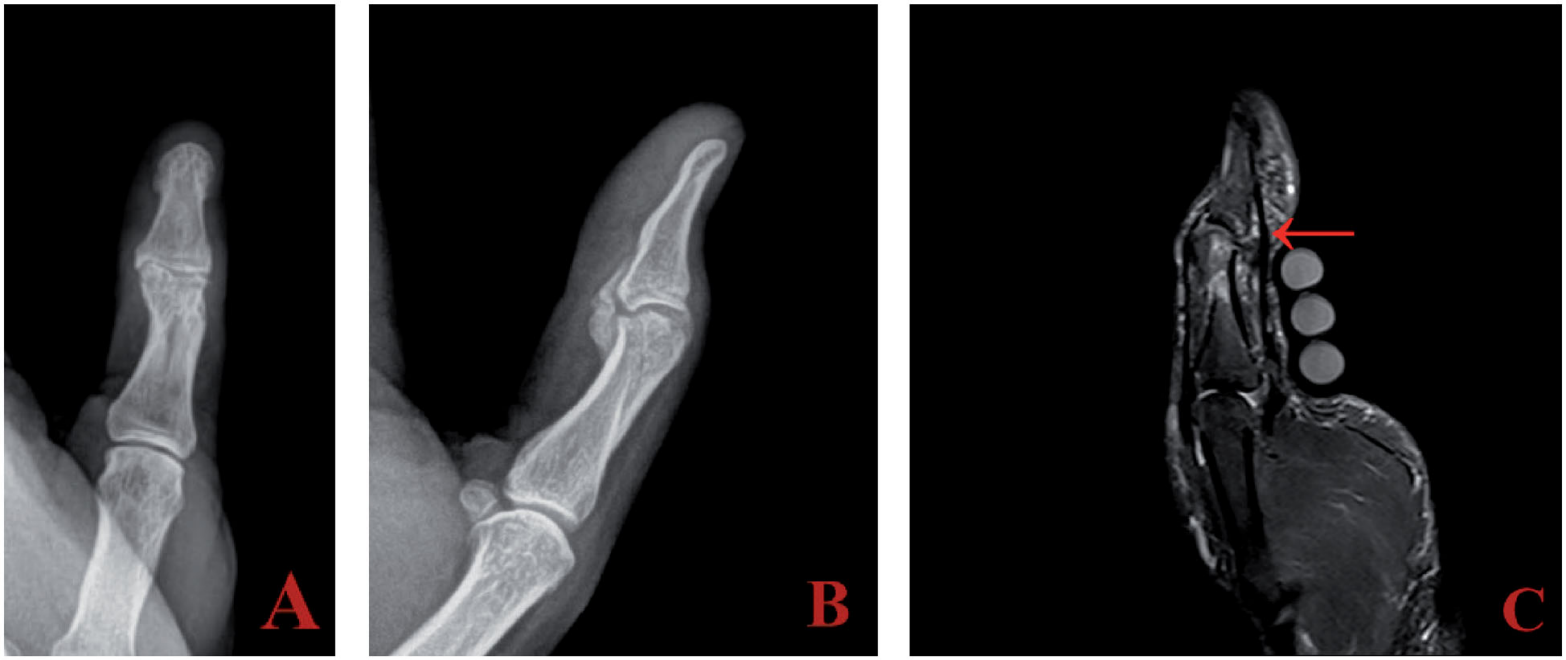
Anteroposterior (A) and lateral (B) views at eight months post injury show HO progression into synostosis. Sagittal STIR image on MRI (C) demonstrates an intact flexor tendon (red arrow) without involvement in synostosis.
